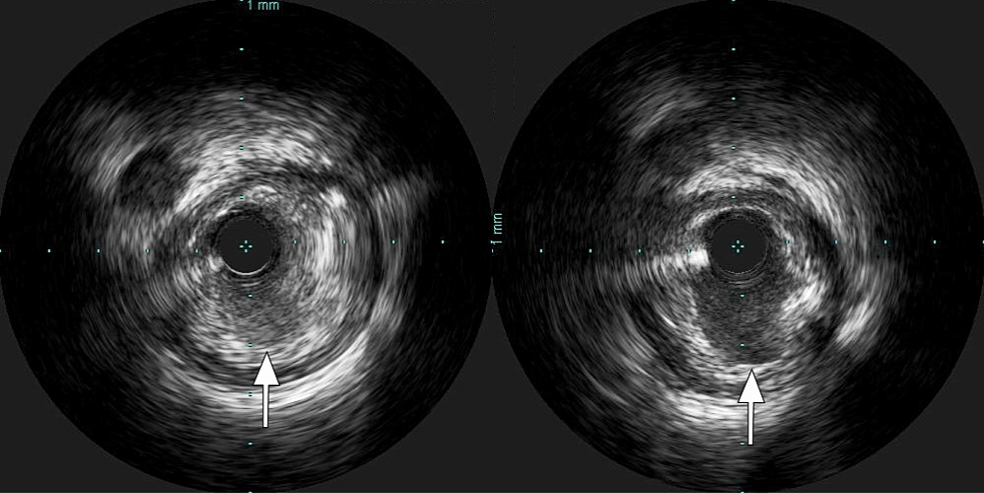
Intravascular ultrasound sonography (Left) Plaque rupture and thrombosis around low echoic plaque in intravascular ultrasonography. The white arrow indicates raptured plaque filled with thrombus. (Right) The white arrow indicates an ulcer discovered after thrombosis aspiration.
